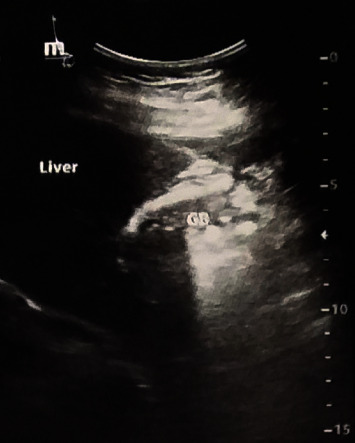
Scleroatrophic (shrunken) gallbladder with a normal biliary tree at abdominal ultrasonography.
